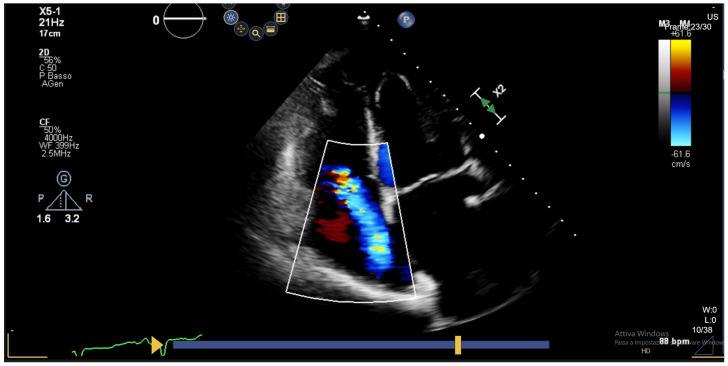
Color Doppler assessment of a severe functional tricuspid regurgitation due to annulus dilatation.
